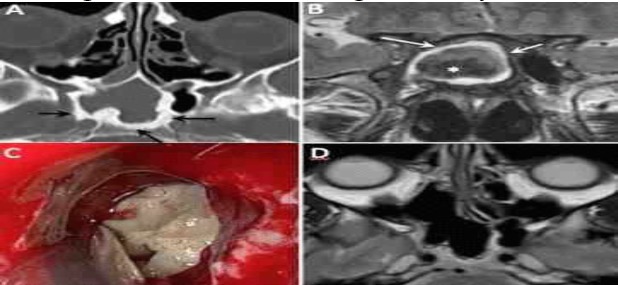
Axial (A) computed tomography scan showing complete opacification and sclerosis of the right sphenoidal walls (reactive osteitis) (black arrows). Coronal T2-weighted magnetic resonance imaging (B) shows isointense signal with associated material filling near signal void (small star). Note also hyperintense signal of thickened inflamed mucosa lining the sinus wall (white arrows). Endoscopic preoperative view of the right sphenoid sinus showing typical friable cheesy yellow to brown material (C). Postoperative axial computed tomography scan showing complete resolution of the right sphenoid disease (D).
